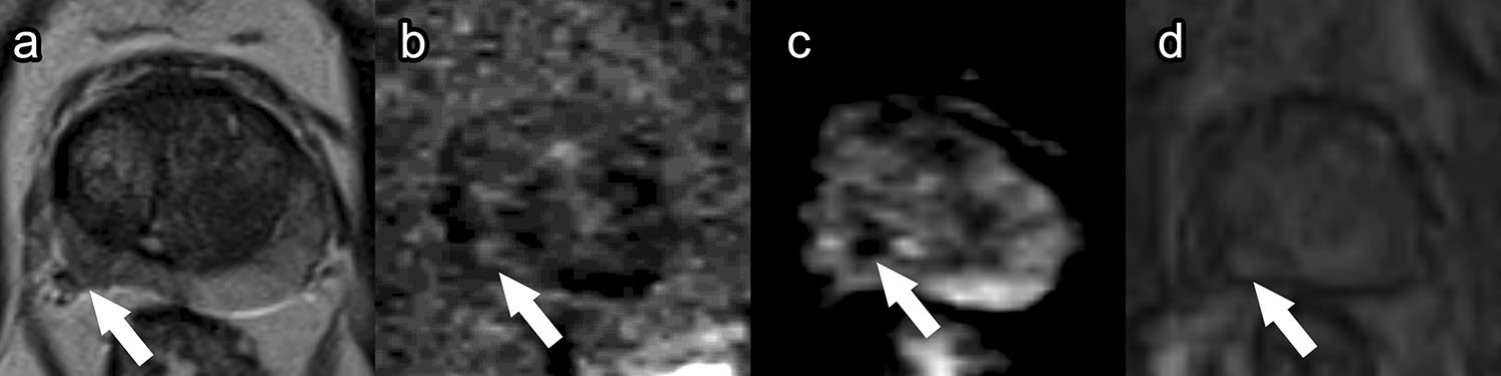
Recurrence in a patient after high-intensity focused ultrasound. Post-treatment prostate MRI: Axial T2 (a), DWI (b), ADC (c), and post contrast T1 (d) demonstrate a right posterolateral peripheral zone lesion with heterogeneous T2 dark signal, restricted diffusion and early arterial hyperenhancement (arrows). Biopsied as Gleason 3 + 4 recurrent prostate cancer with perineurial invasion
